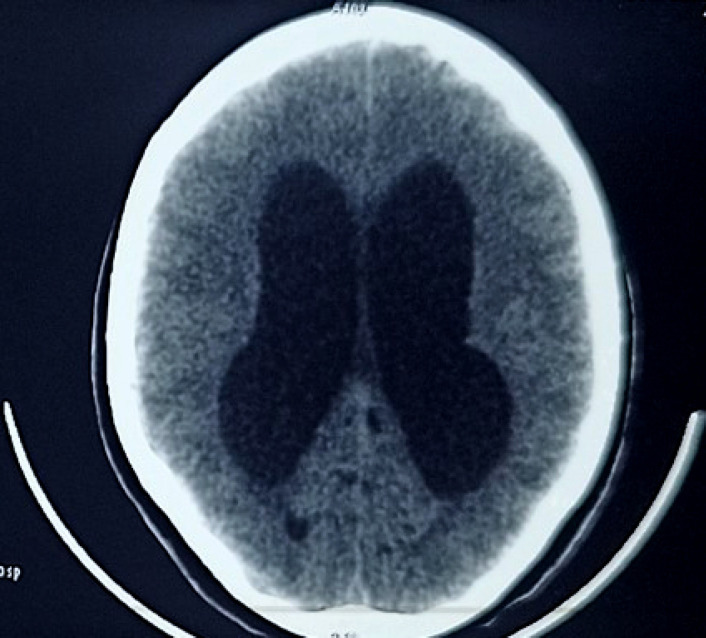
Tomodensitométrie cérébrale en coupe axiale avant injection de produit de contraste: dilatation tétraventriculaire associée à des hypodensités diffuses de la substance blanche chez une patiente de 18 ans séronégative pour le VIH, atteinte de cryptococcose neuroméningée et tuberculose pulmonaire Cerebral CT scan in axial section before injection of contrast medium: tetraventricular dilation associated with diffuse white matter hypodensity in an 18-year-old HIV-negative patient with neuromeningeal cryptococcosis and pulmonary tuberculosis
